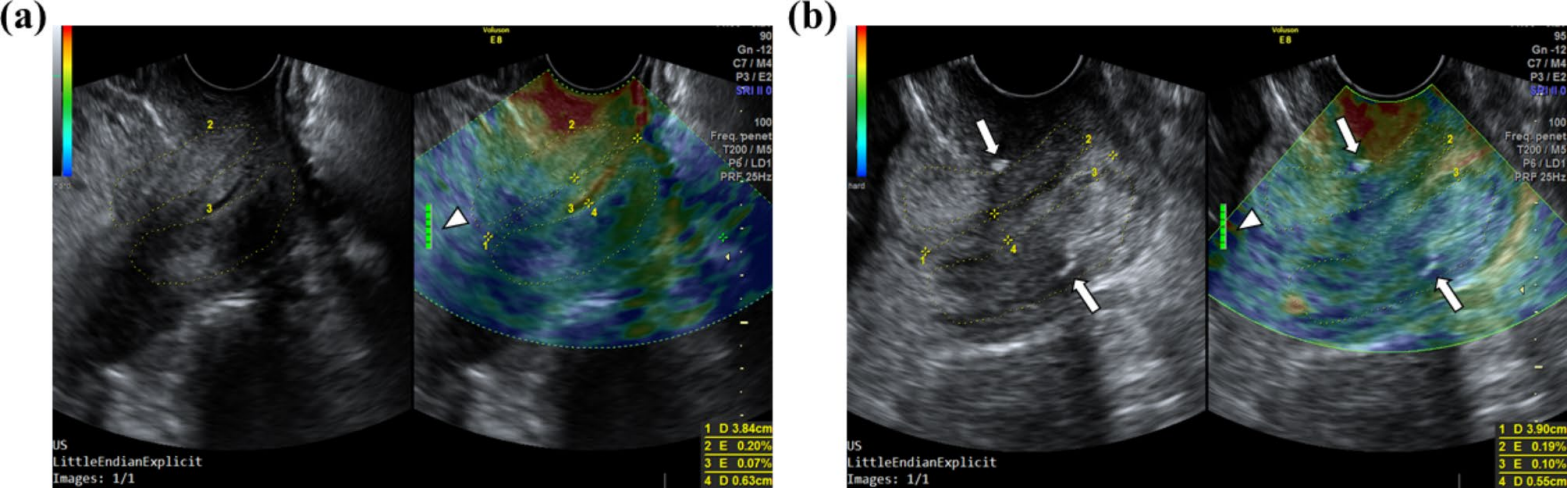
Dual-mode grayscale sonographic images (left) and real-time elastograms (right) of an insufficient cervix in the first trimester (a) and the second trimester (b). Elastograms showed differences in the stiffness of anterior and posterior cervical lips visualized as different colors, with red, blue, and green indicating soft, hard, and intermediate tissues, respectively. The cervical length and endocervical canal width were also measured. Full green quality bar was achieved after appropriate manual compression (arrowhead). Cerclage stiches are shown in the second trimester (arrow)
